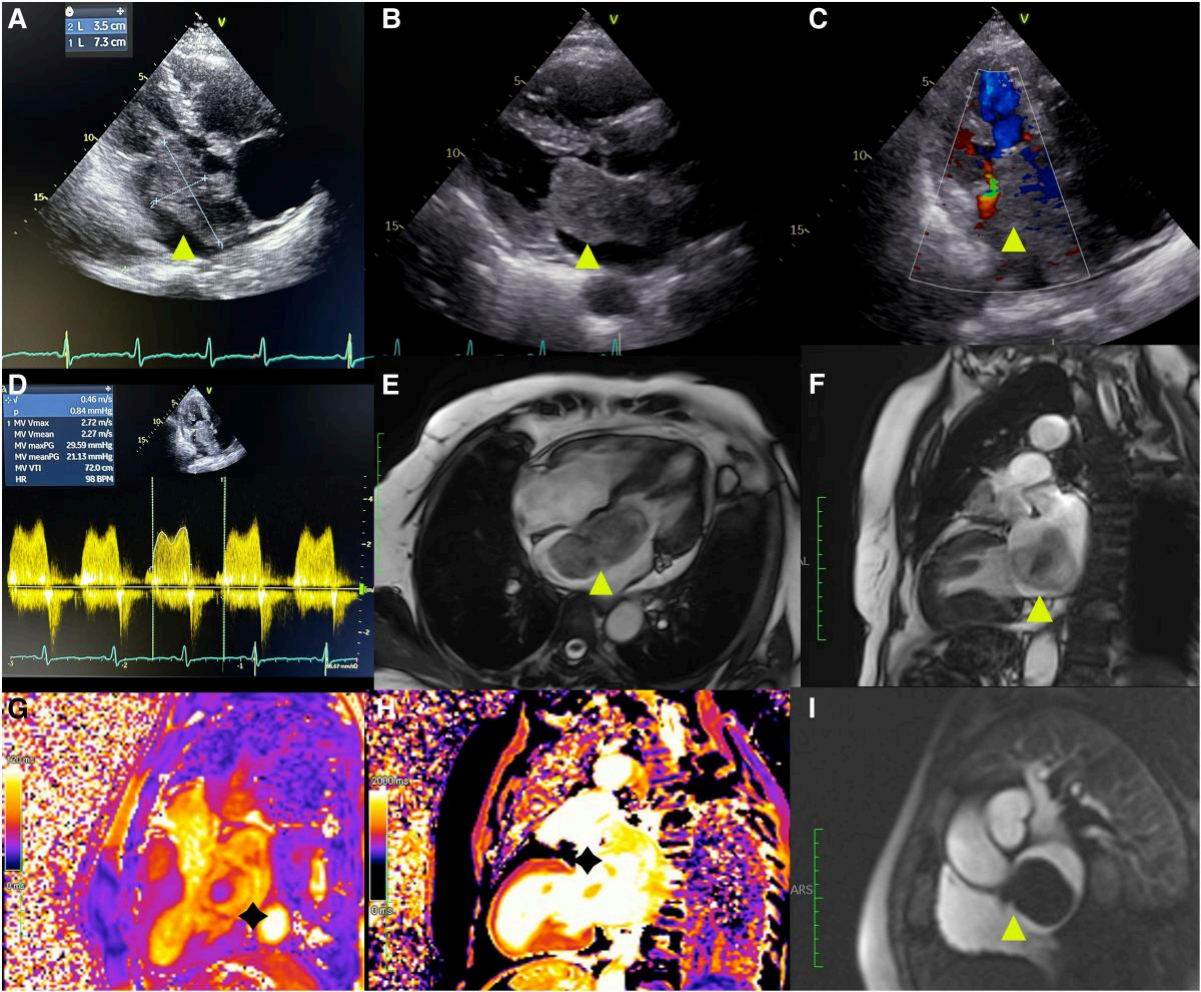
Echocardiography demonstrates a hyperechoic polypoid mass (arrowhead) obstructing the mitral valve in the (*A*) apical four-chamber, (*B*) parasternal long-axis, and (*C*) apical three-chamber views. (*D*) MV CW Doppler imaging shows an increased gradient (maximum MVG: 29 mmHg, mean MVG: 21 mmHg) due to the mass obstruction. Cardiac MRI demonstrates a mass with high signal intensity and heterogenous components on the horizontal long-axis gradient echo sequence: (*E*) four-chamber and (*F*) two-chamber views. In the T1 and T2 mapping sequences, a cystic mass (asterisk) was demonstrated with increased (*G*) T2 time and (*H*) native T1 value. (*I*) Turbo Flash sequence demonstrated the mass effect of the cystic lesion causing mitral valve obstruction. CW, continuous wave; MV, mitral valve; MVG, MV gradient; VTI, velocity time integral.
